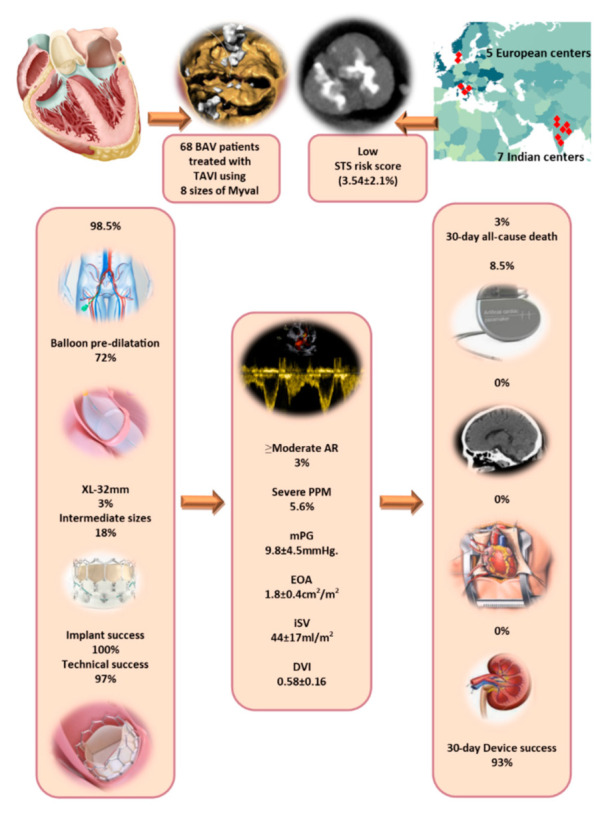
Visual abstract of the Myval bicuspid registry summarizing the key findings of the registry; including the procedural details and outcome, 30-day echocardiographic and clinical outcomes. AR: Aortic regurgitation; iSV: indexed stroke volume; mPG: mean pressure gradient; PPM: patient prosthesis mismatch; DVI: dimensionless velocity index. EOA: Effecive orifice area; BAV: Bicuspid aortic valve STS: Society of Thoracic Surgeons; TAVI: Transcatheter aortic valve implanttaion.
